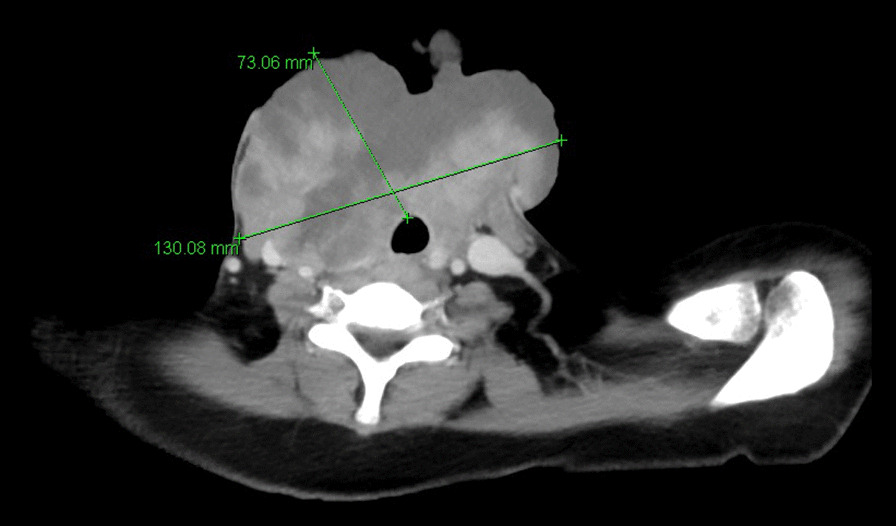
Preoperative computed tomography of the neck with a widespread (130 × 73 mm) inhomogeneous mass in the thyroid lodge
